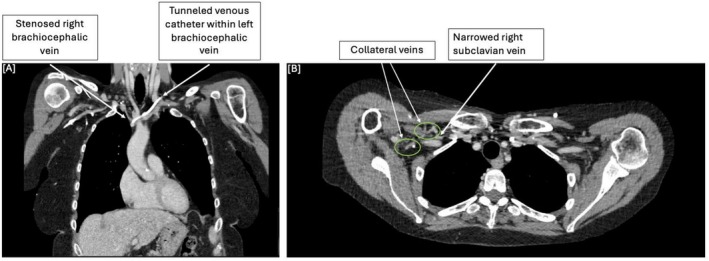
CT venography demonstrating central venous stenosis with collateralisation. (A) Coronal plane showing the current position of the catheter within the left brachiocephalic vein and stenosis of the right brachiocephalic vein. (B) Transverse plane showing stenosis of the right subclavian vein with formation of collateral veins.
